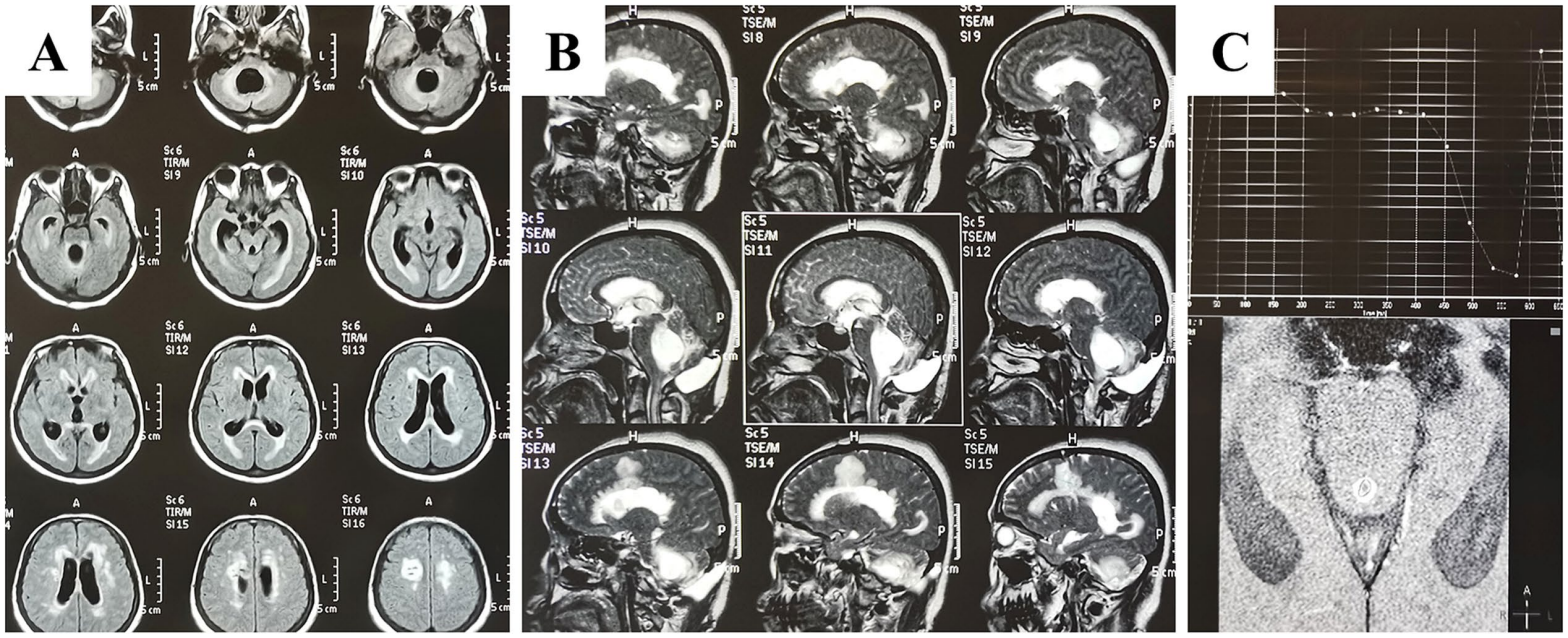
Pre-operative MRI images. (A) Axial MRI in T2 Flair scans displaying dilated bilateral cerebral ventricles, third ventricle, midbrain aqueduct, and fourth ventricle, accompanied by interstitial edema. (B) Sagittal MRI in T2 phase showing the dilated ventricles and interstitial edema. (C) Phase-contrast cine MRI of cerebrospinal fluid demonstrating an obstruction in the midbrain aqueduct. *Abbreviations:* MRI, magnetic resonance imaging; Flair, fluid-attenuated inversion recovery.
